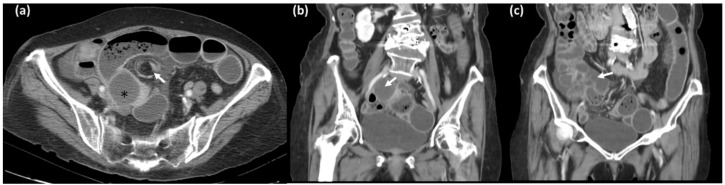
Three months later she presented to the emergency department again with abdominal pain and distension, they repeated the contrast-enhanced computed tomography of abdomen–pelvis: (a) Dilated small bowel loops, with whirl sign (arrow), which may indicate bowel volvulus or closed loop obstruction. A cystic structure adjacent to transition zone was found (*) suspected to be the enlarged appendix. (arrows in coronal image (b,c)) [1,2].
